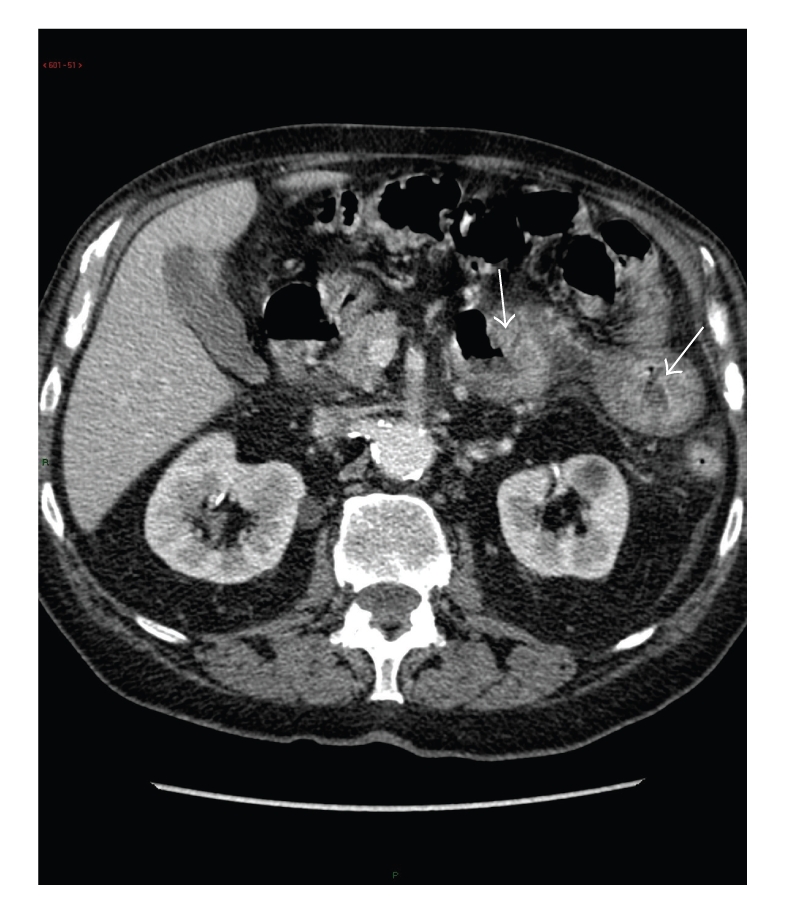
Abdomen computed tomography after intravenous contrast. Significant thickening of the Treitz angle and proximal jejunum (arrows), with infiltration of the loco-regional fat.
